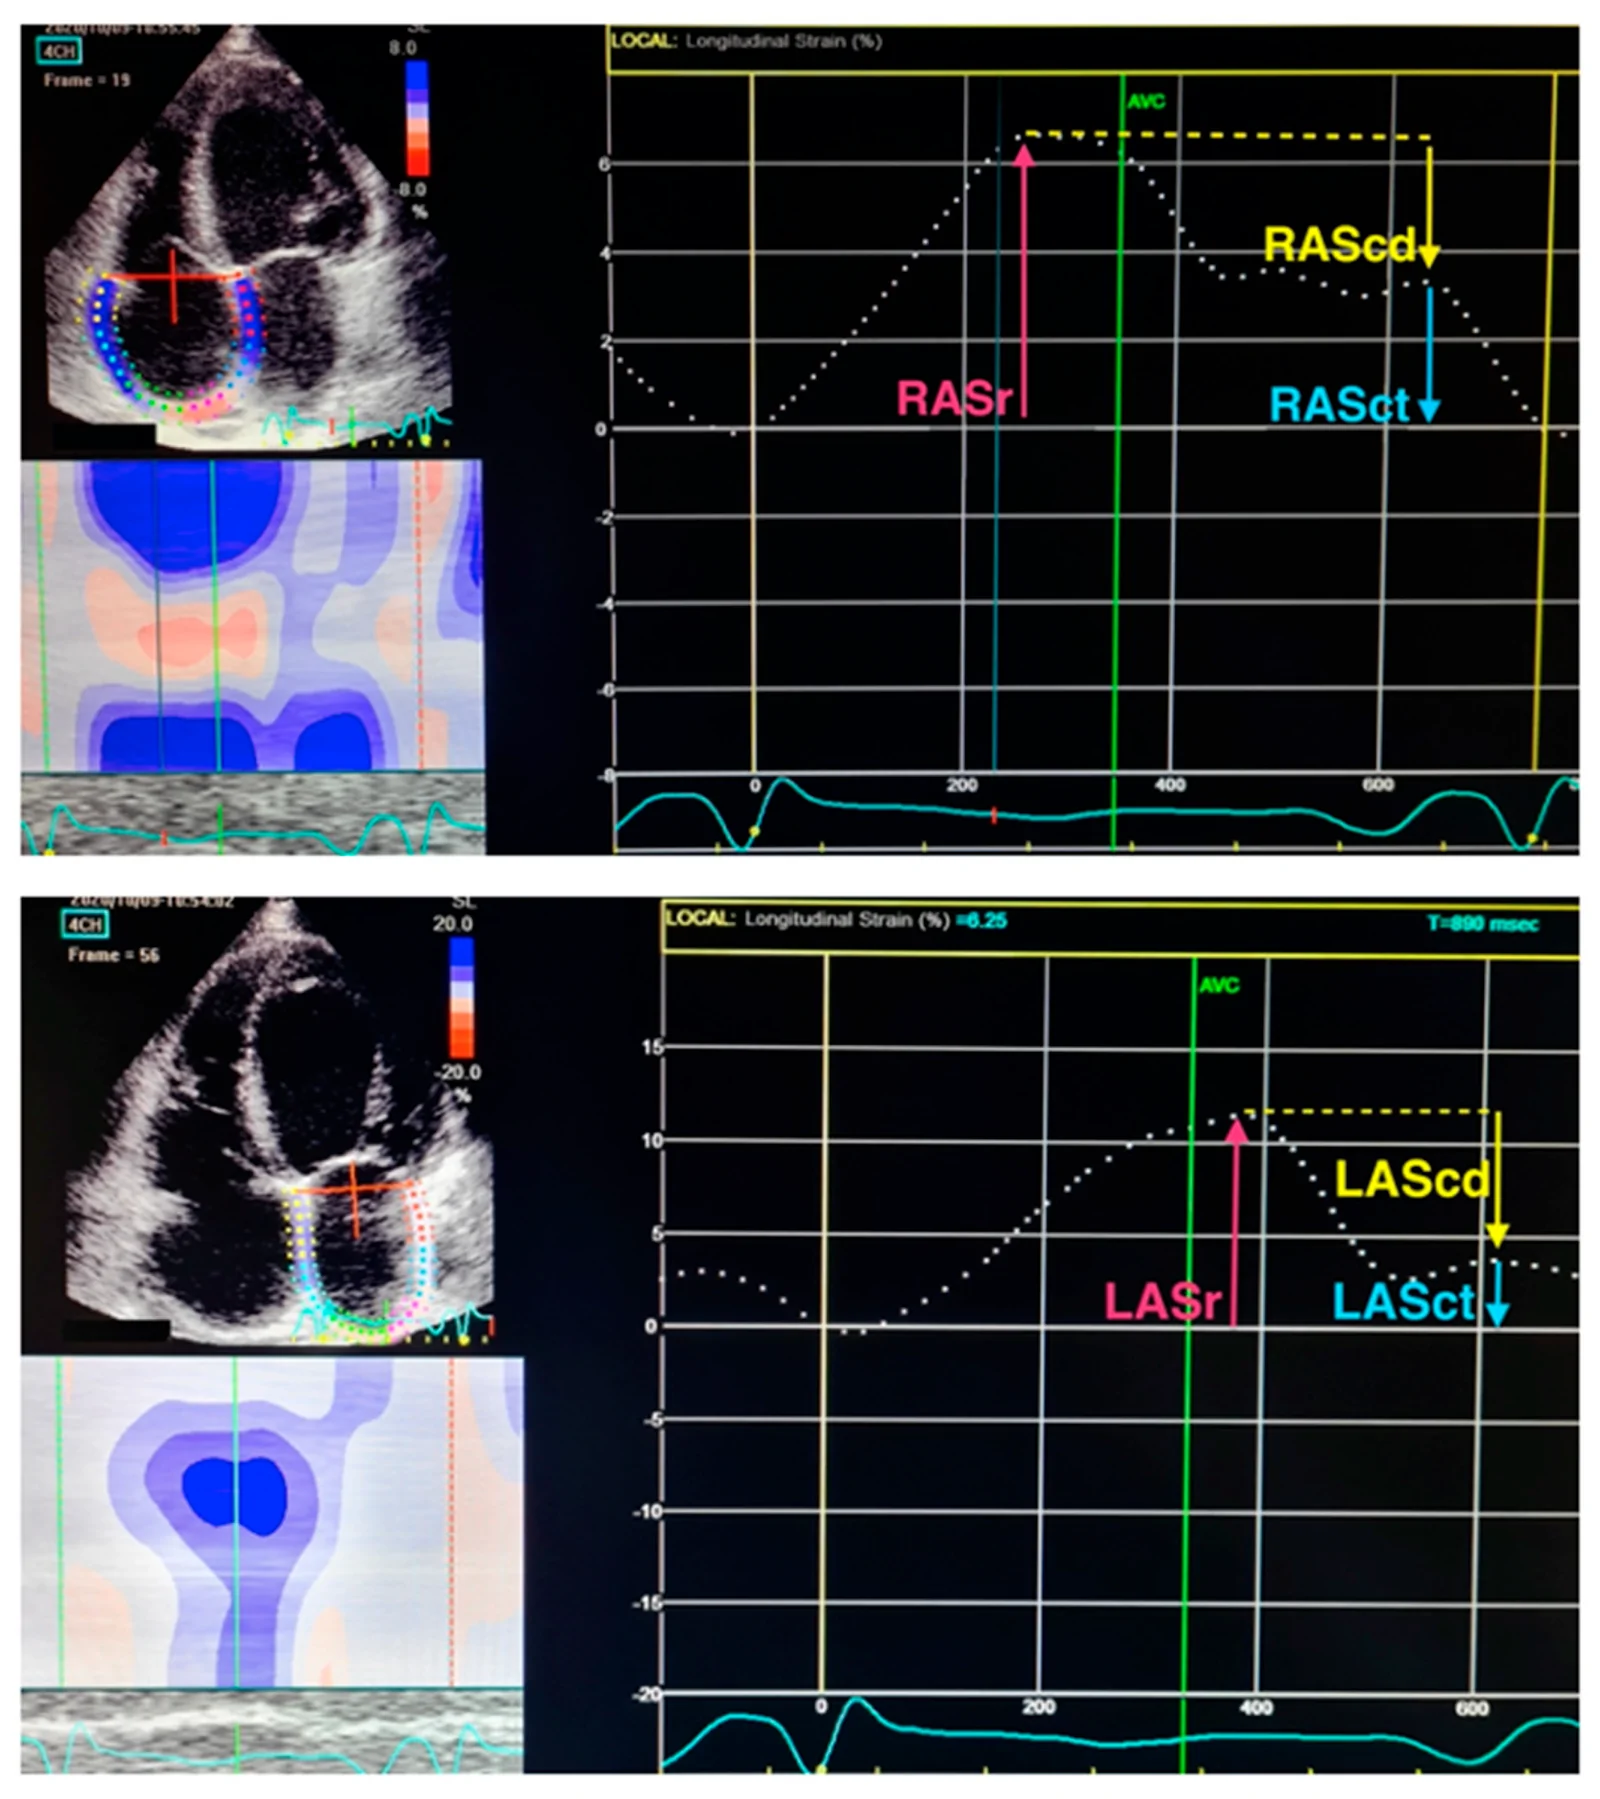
Apical four-chamber view with the average strain curve for the right atrium (upper panel) and left atrium (lower panel), used to measure reservoir strain (positive value), conduit and contraction strain (negative values). RASr—right atrial reservoir strain; RAScd—right atrial conduit strain; RASct—right atrial contraction strain; LASr—left atrial reservoir strain; LAScd—left atrial conduit strain; LASct—left atrial contraction strain.
